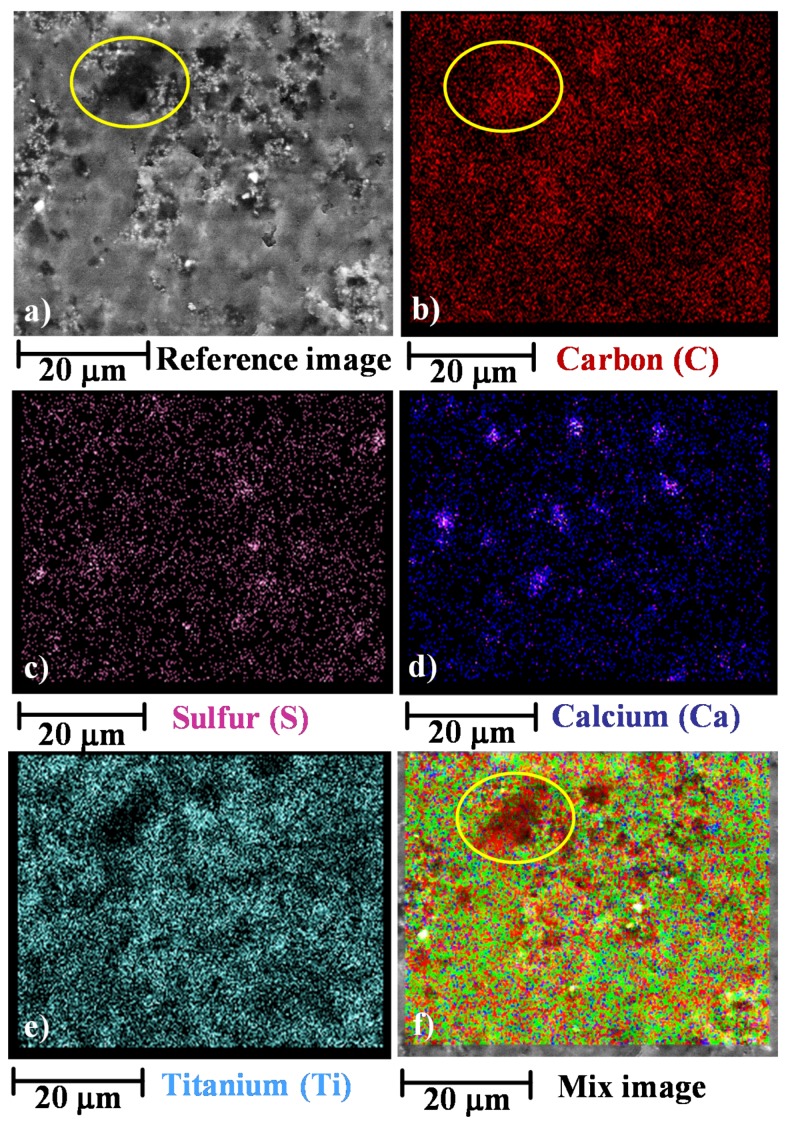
Energy dispersive X-ray (EDX) performed on the fracture surface of the sample 01GNPs: (a) reference image; (b) map distribution of C; (c) map distribution of S; (d) map distribution of Ca; (e) map distribution of Ti; (f) mix image.
